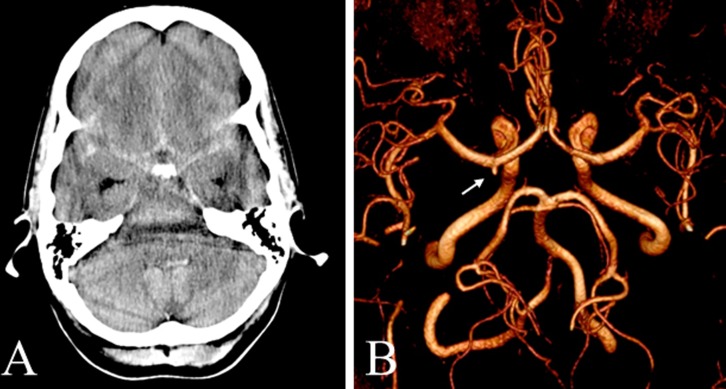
Initially undetected aneurysm found 8 years after SAH of a 44-year-old female patient (Table 1, #21). *A* CT scan at admission in 2005 revealing nSAH in the frontal interhemispheric fissure, in the premedullary cisterns and the bilateral sylvian fissures. The consecutive digital subtraction angiography was false negative. *B* Intracranial TOF-angiogram of the same patient in July 2013 demonstrating an aneurysm of the right internal carotid artery *(arrow)* which was retrospectively visible in the initial post-hemorrhagic digital subtraction angiography (Fig. 3).
